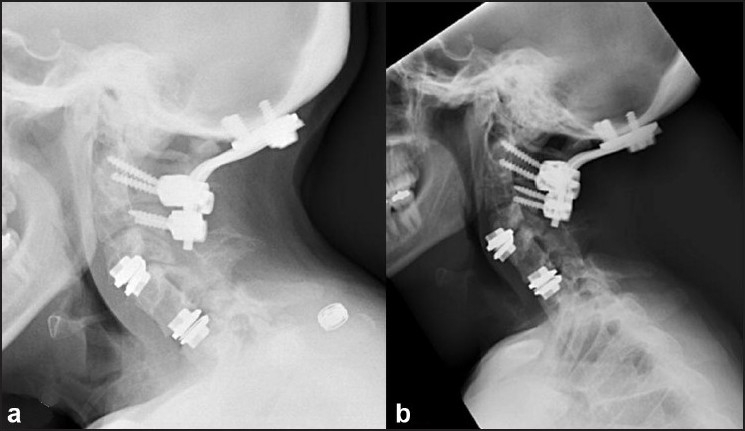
Immediate postoperative lateral radiograph (a) demonstrating two-level arthroplasty. 5 months follow-up lateral radiograph (b) shows extrusion and anterior migration of the C4-5 prosthesis
